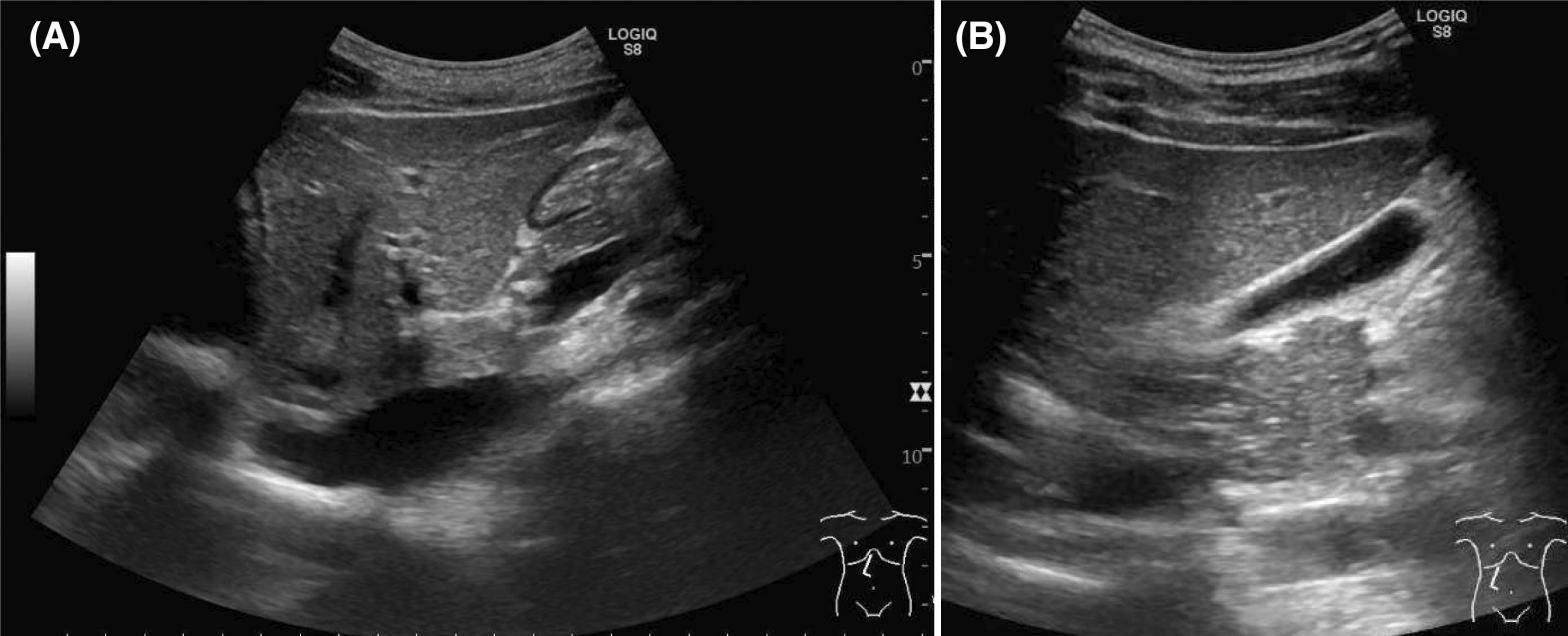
Abdominal ultrasonography. Abdominal ultrasonography showed no specific change.
